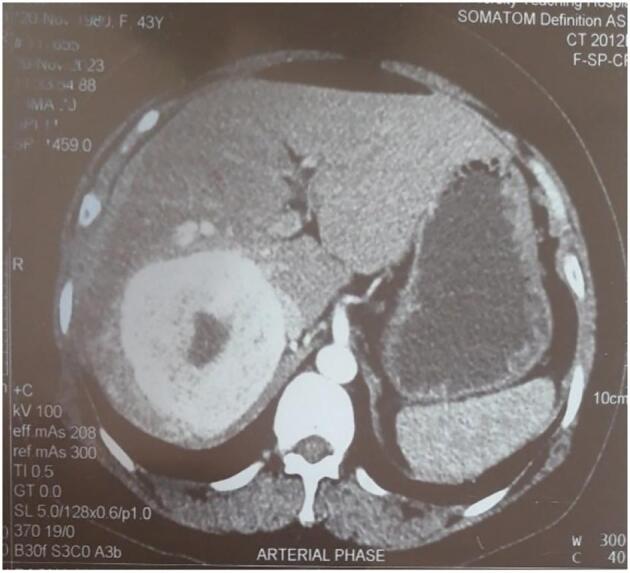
a: Imaging finding (CT scan) of the liver mass which showed a well-defined hypodense lesion in the right lobe of the liver, with a differential diagnosis of focal nodular hyperplasia.
